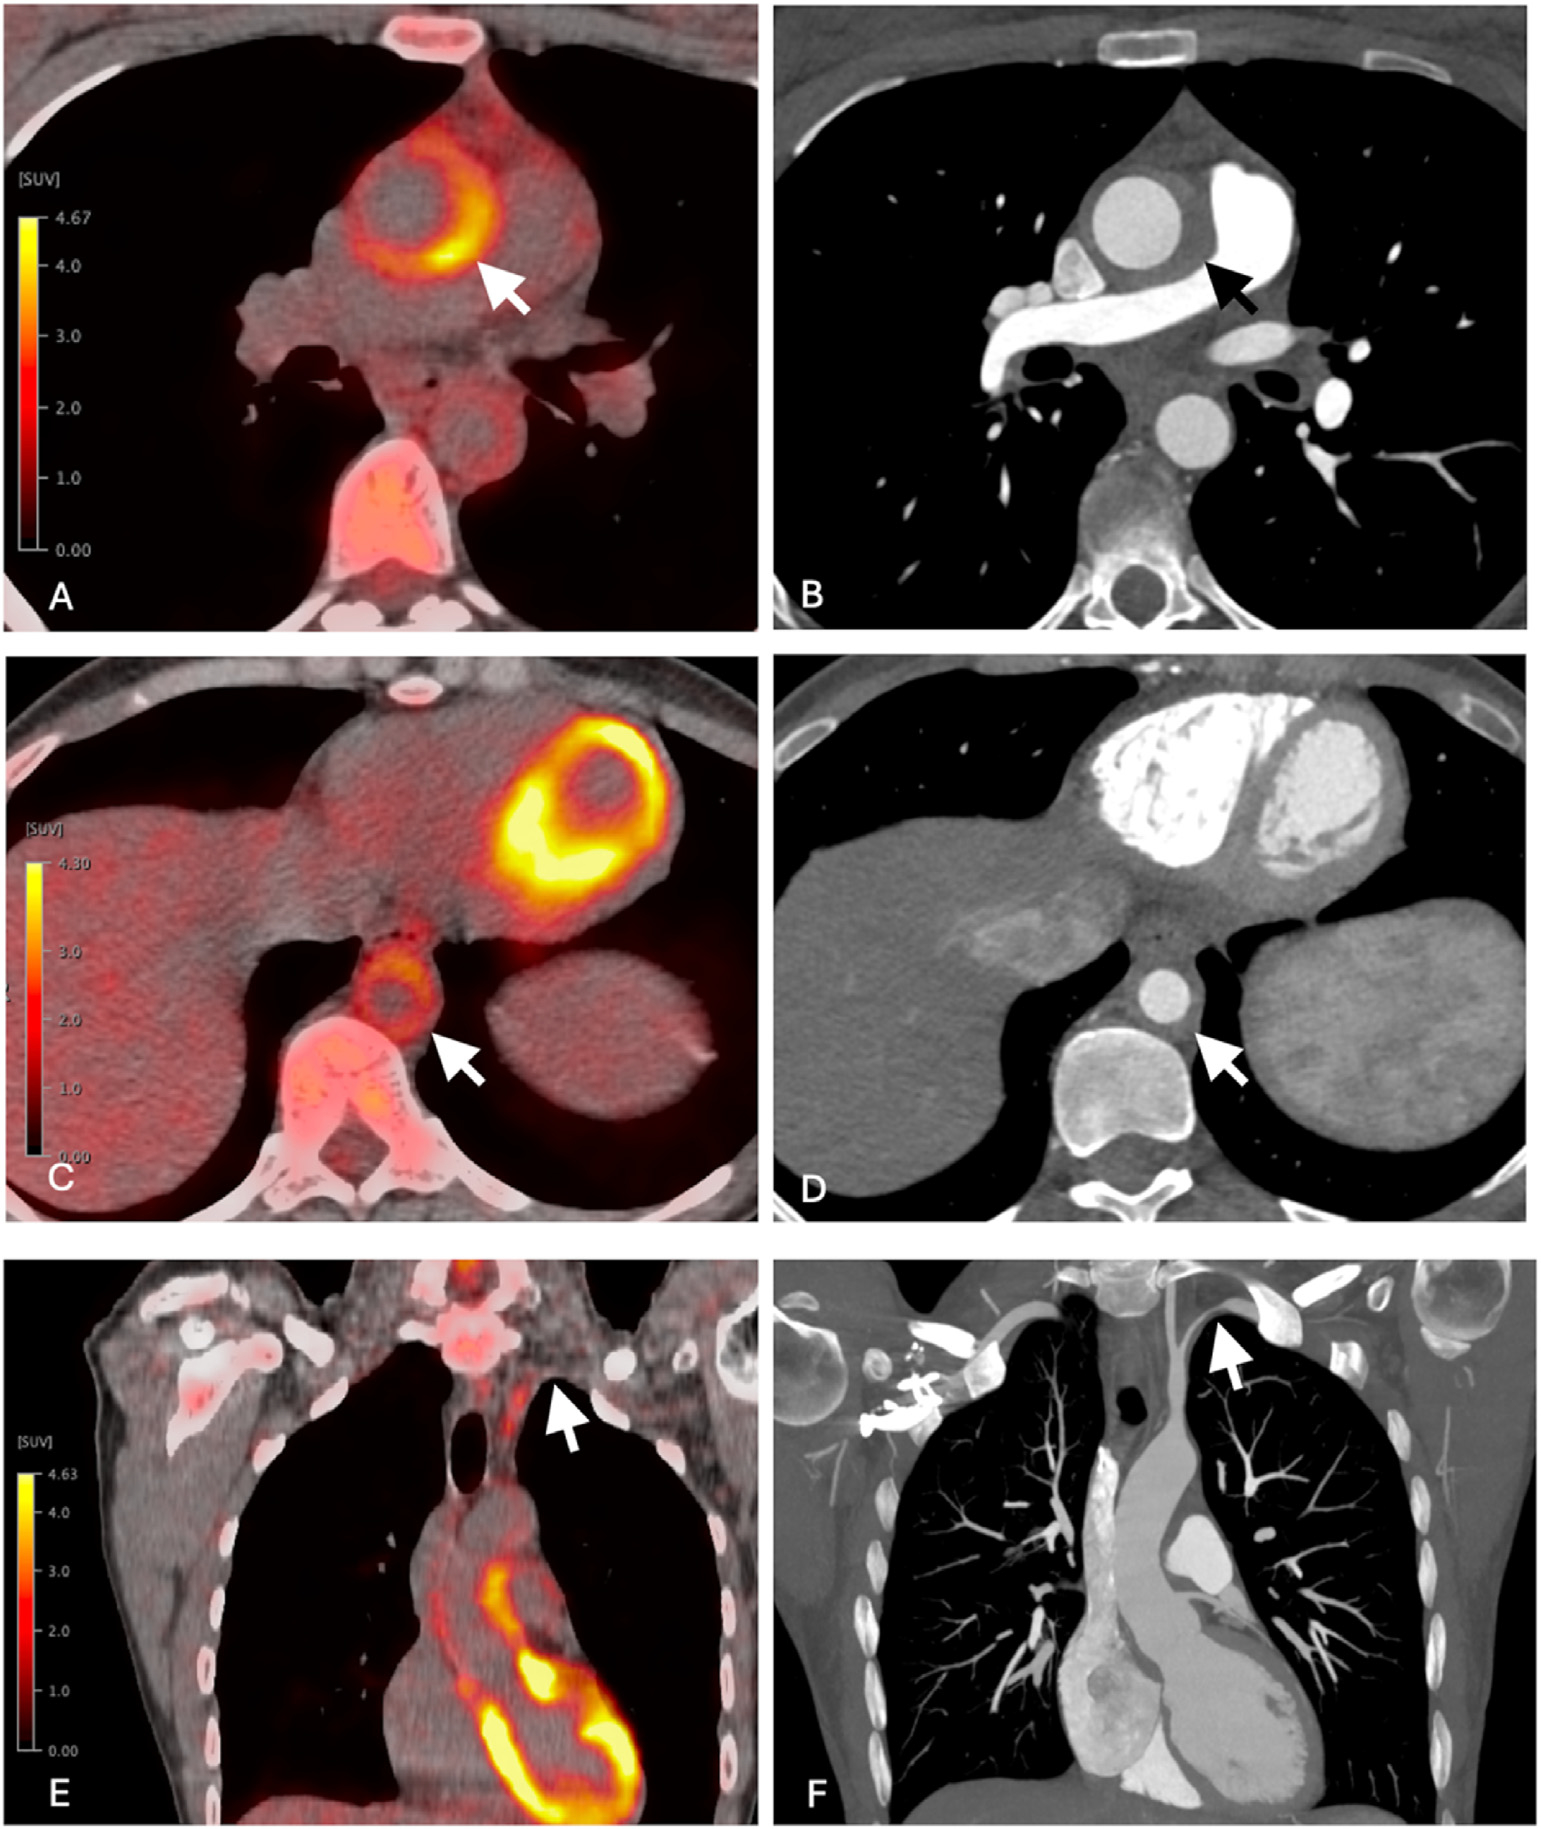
35-year-old female with a remote history of TAK presented with fatigue and elevated inflammatory markers. Given the expected chronic changes in the vessels, the decision has been made to proceed with FDG-PET/CTA to assess disease activity along with vascular complications. (A) Axial FDG-PET/CT image of the ascending aorta and (C) the descending aorta demonstrate circumferentially intense FDG uptake of the wall (arrows), corresponding to thickening (B,D) on axial CTA image (arrows). These findings are consistent with a relapse/active vasculitis. However, the coronal FDG-PET/CT image (E) of the chest, shows none-to-mild FDG uptake of the left subclavian artery (arrow), corresponding to severe narrowing (arrow) of the vessel on coronal MIP CTA image (F), most likely represents the sequela of prior vasculitis. TAK, Takayasu’s arteritis; FDG-PET/CTA, 18F]-fluoro-2-deoxy-d-glucose positron emission tomography/computed tomography angiography.
